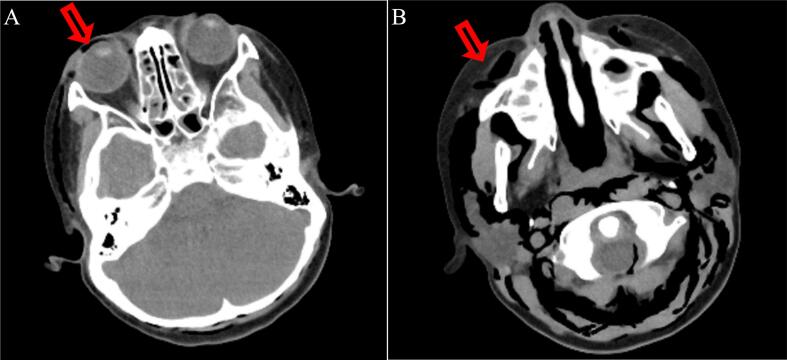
A. Axial computed tomography of facial bones showing orbital emphysema (red arrow). B. Axial computed tomography of facial bones showing subcutaneous periorbital emphysema (red arrow).
